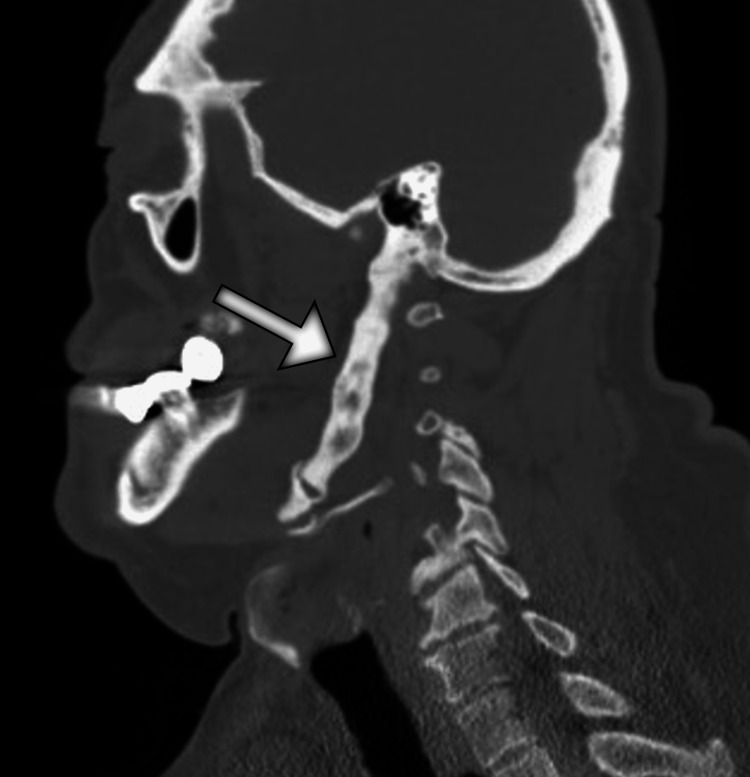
Sagittal CT image of the neck shows a markedly thickened and prolonged left styloid process (arrow) that reaches the hyoid bone. CT: computed tomography
